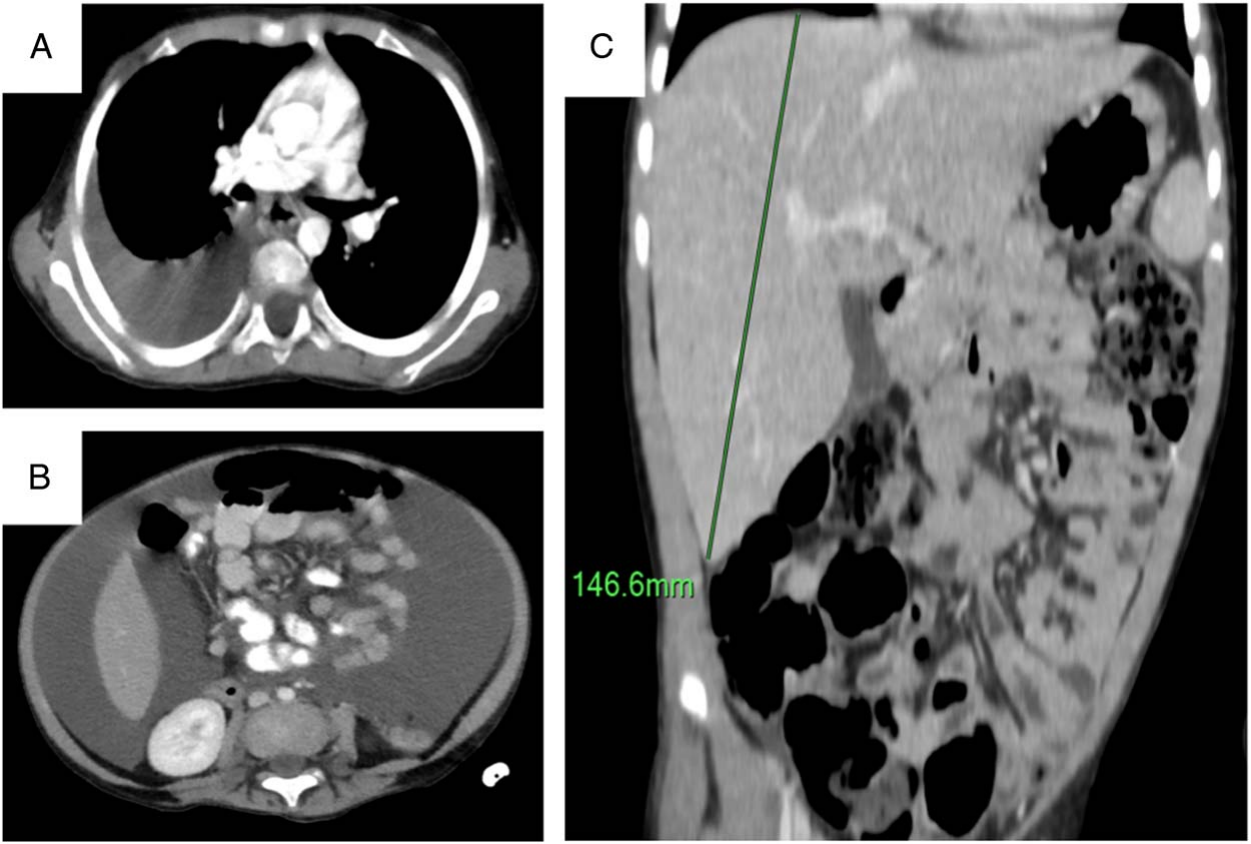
Computed tomography scan of (A) the chest showing right-sided pleural effusion, (B) the abdomen showing massive amounts of ascitic fluid, and (C) the abdomen showing hepatomegaly.
